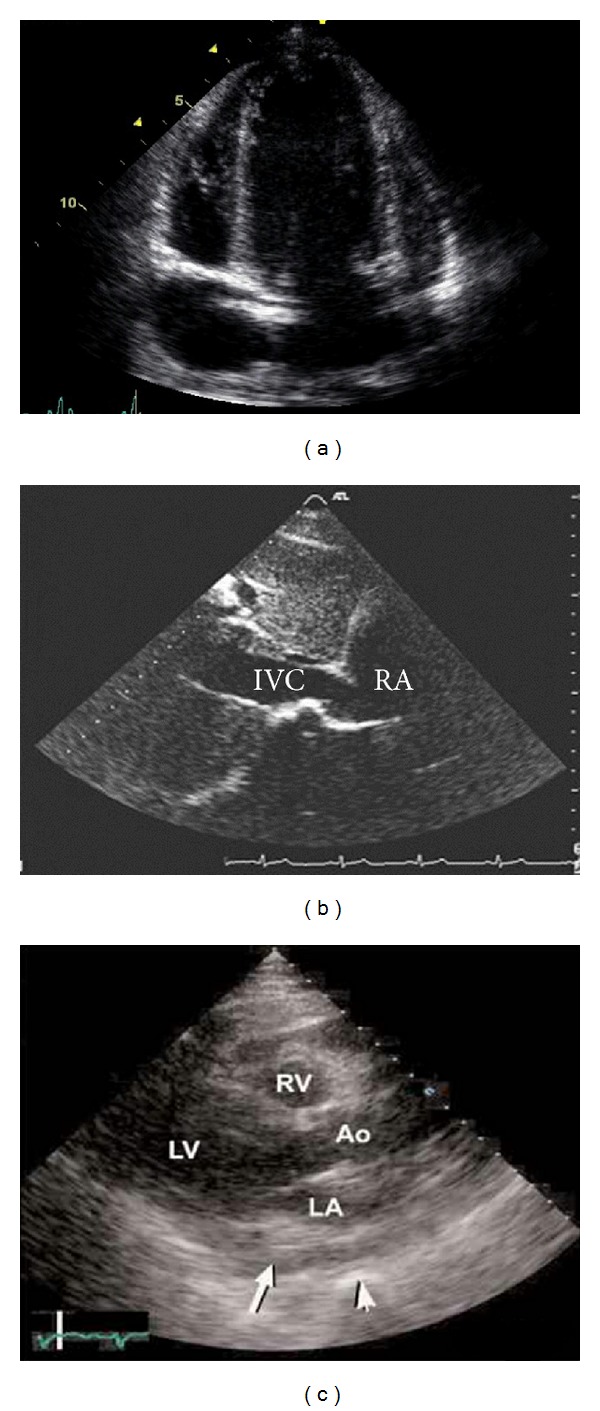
Echocardiography showing septal bouncing (a), dilation of IVC (b), pericardial effusion, and calcification (c).
